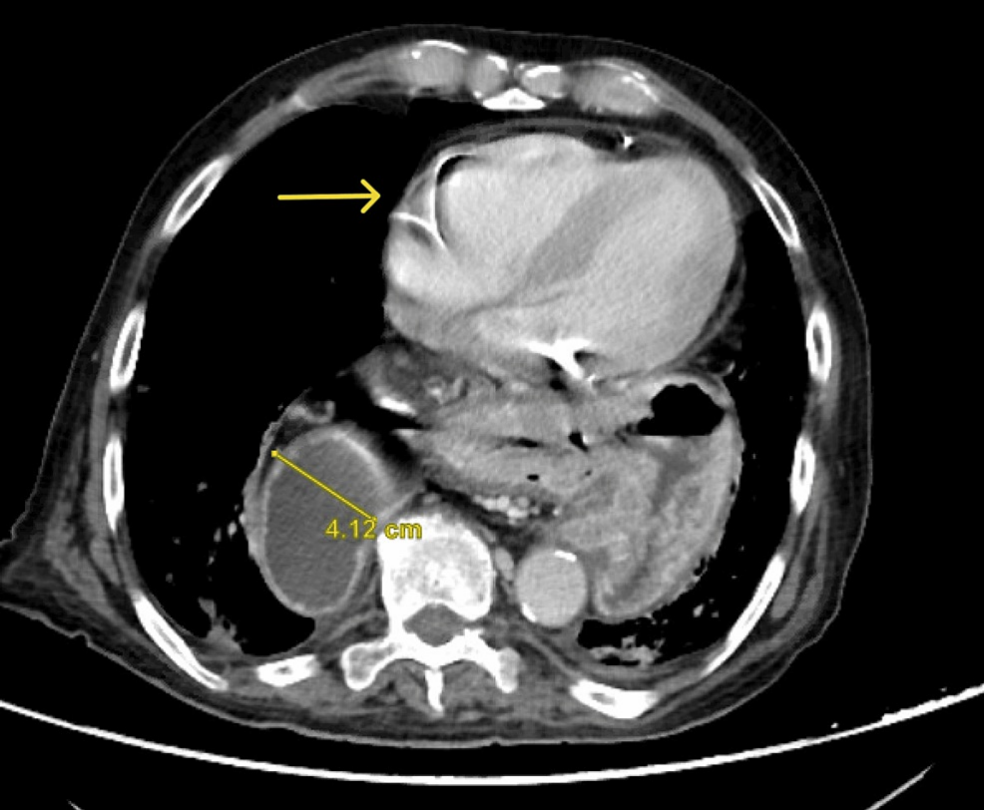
Abdominal CT with contrast (axial view) The image shows a large hiatal hernia containing the entire stomach and part of the duodenum (arrow) with an abrupt transition zone at the duodenum.
